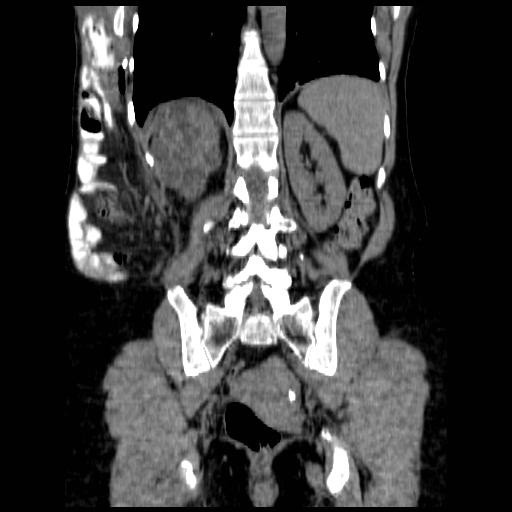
Coronal CT scan demonstrating incisional lumbar hernia.
